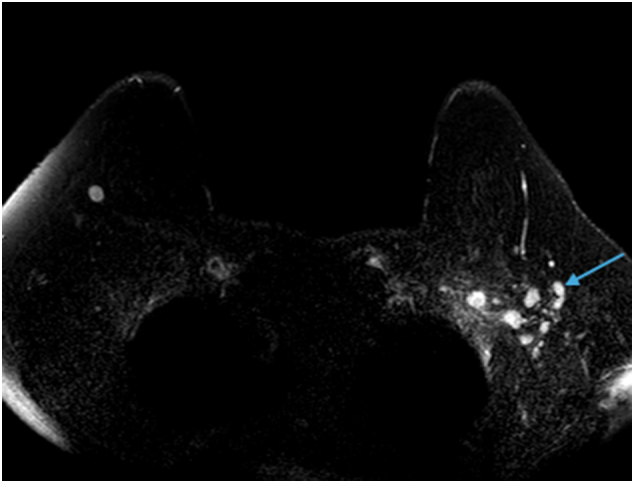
MRI showed left axillary lymph nodes (arrow), twenty-nine days after second COVID-19 vaccination.
